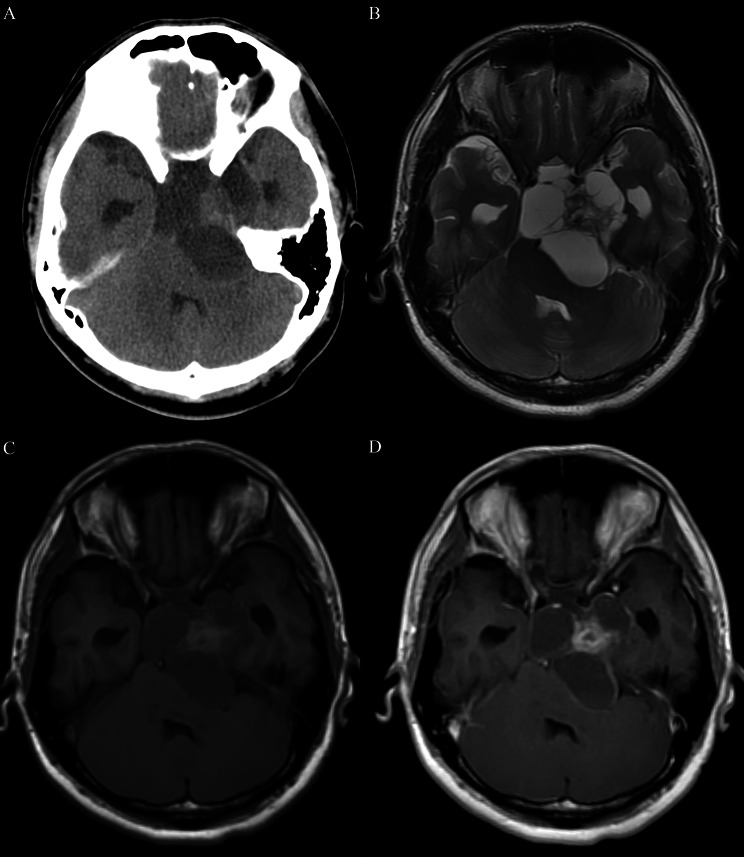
A 23-year-old patient with a suprasellar PA. (A) Axial CT image shows a cystic-solid heterogeneous suprasellar mass. (B) Axial T2-weighted image demonstrates predominant hyperintensity of the mass, with slight hypointensity of the nodule. (C) Axial T1-weighted image reveals hypointensity of the mass, with slight hyperintensity of the nodule. (D) Axial postcontrast T1-weighted image shows irregular rim enhancement with a mural nodule and multiple crests connecting the indentations on the surface, which is similar to the appearance of the flesh of a cut green pepper
